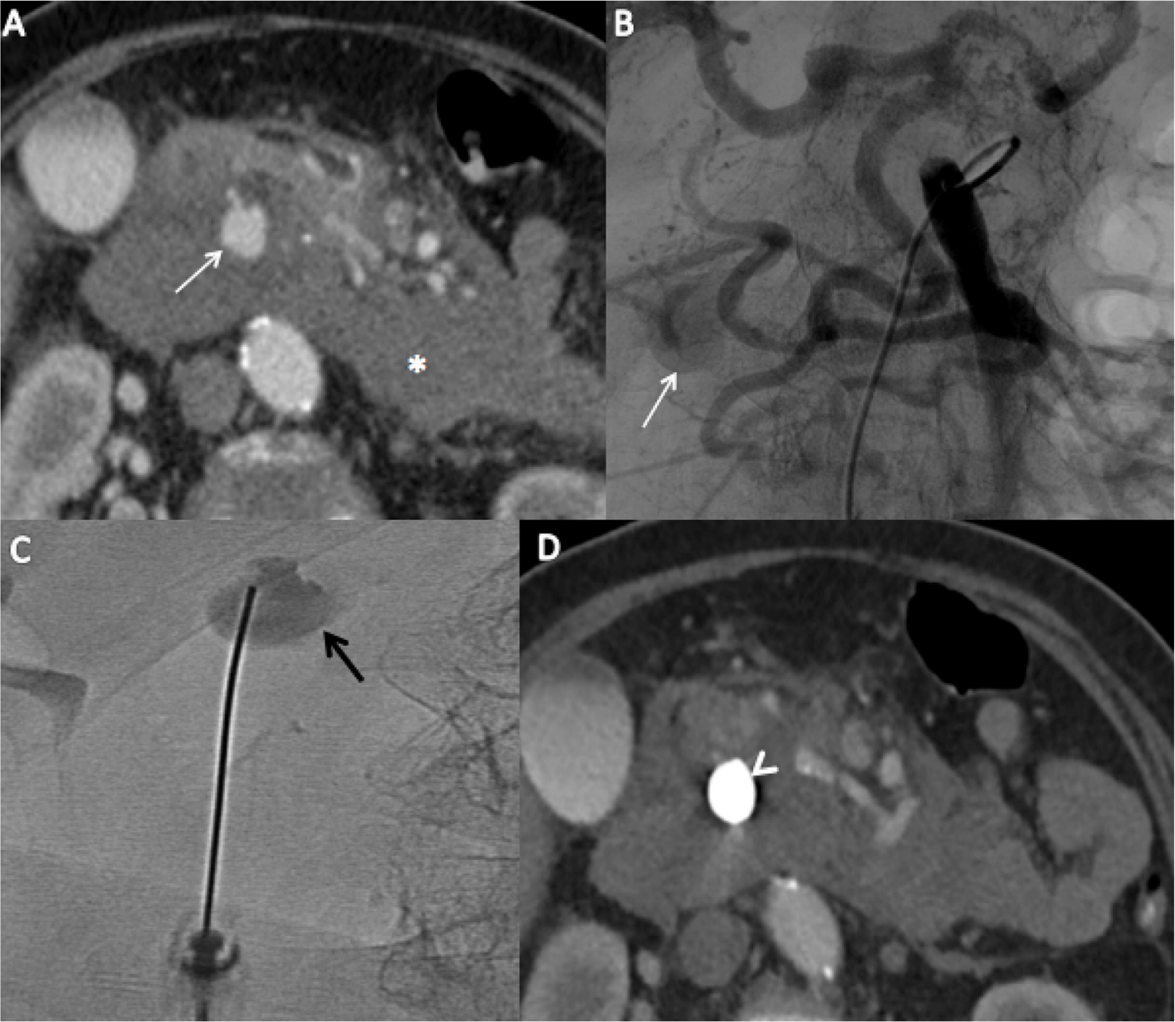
A 74-year-old female with spontaneous mesenteric haematoma following a median arcuate ligament syndrome. Axial CT scan (A) showed a pseudoaneurysm without contrast extravasation of a pancreaticoduodenal artery, with mesenteric hematoma (*). Upper mesenteric angiography (B) showed a prominent gastroduodenal artery that feeds the common hepatic artery and confirmed a pseudoaneurysm of 9 mm, without possibility to catheterize the feeding artery (endovascular technical failure). After puncture under ultrasonographic guidance, a fluoroscopic image (C) showed filling of the pseudoaneurysm using contrast agent injection (black arrow) with a 22G-needle. Post embolization contrast-enhanced axial CT scan (D) showed the absence of filling of the pseudoaneurysm (white arrow’s head)
